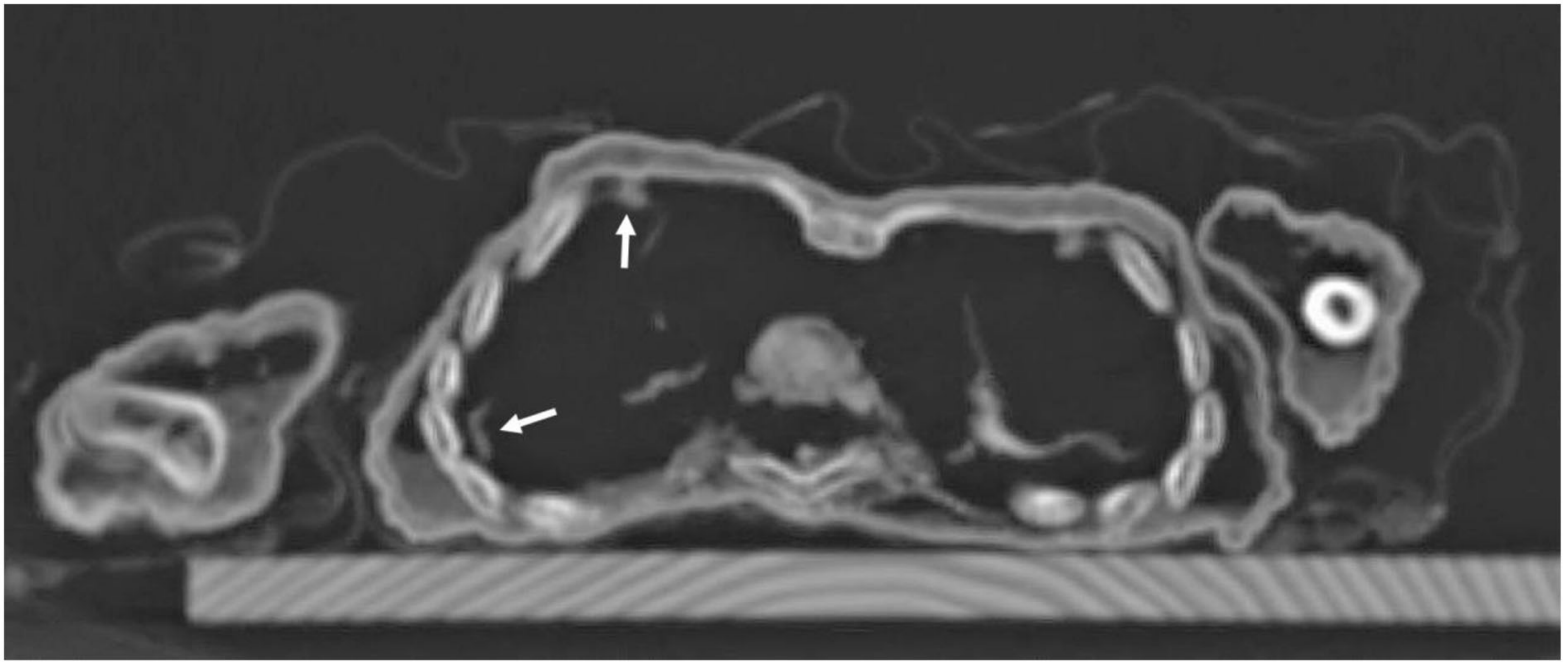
Axial CT sections through the chest: besides the regular skeletal elements, there are remnants of lung tissue in the right chest cavity with some pleural adhesions (arrows).
